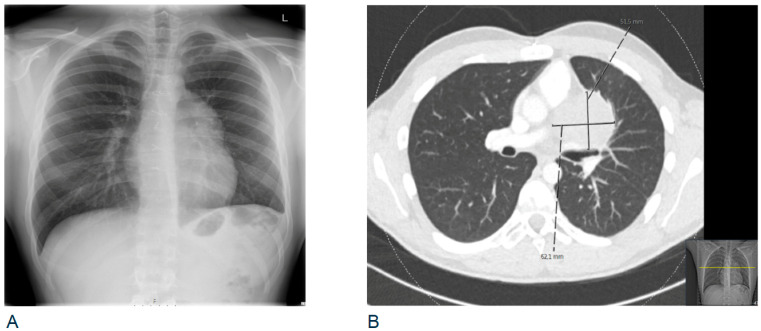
(A) Follow-up chest X-ray showing a broadened aorto-pulmonary window a year and a half after resection of malignant mediastinal GCT; (B) CT scan confirming recurrent mass in the anterior mediastinum.
